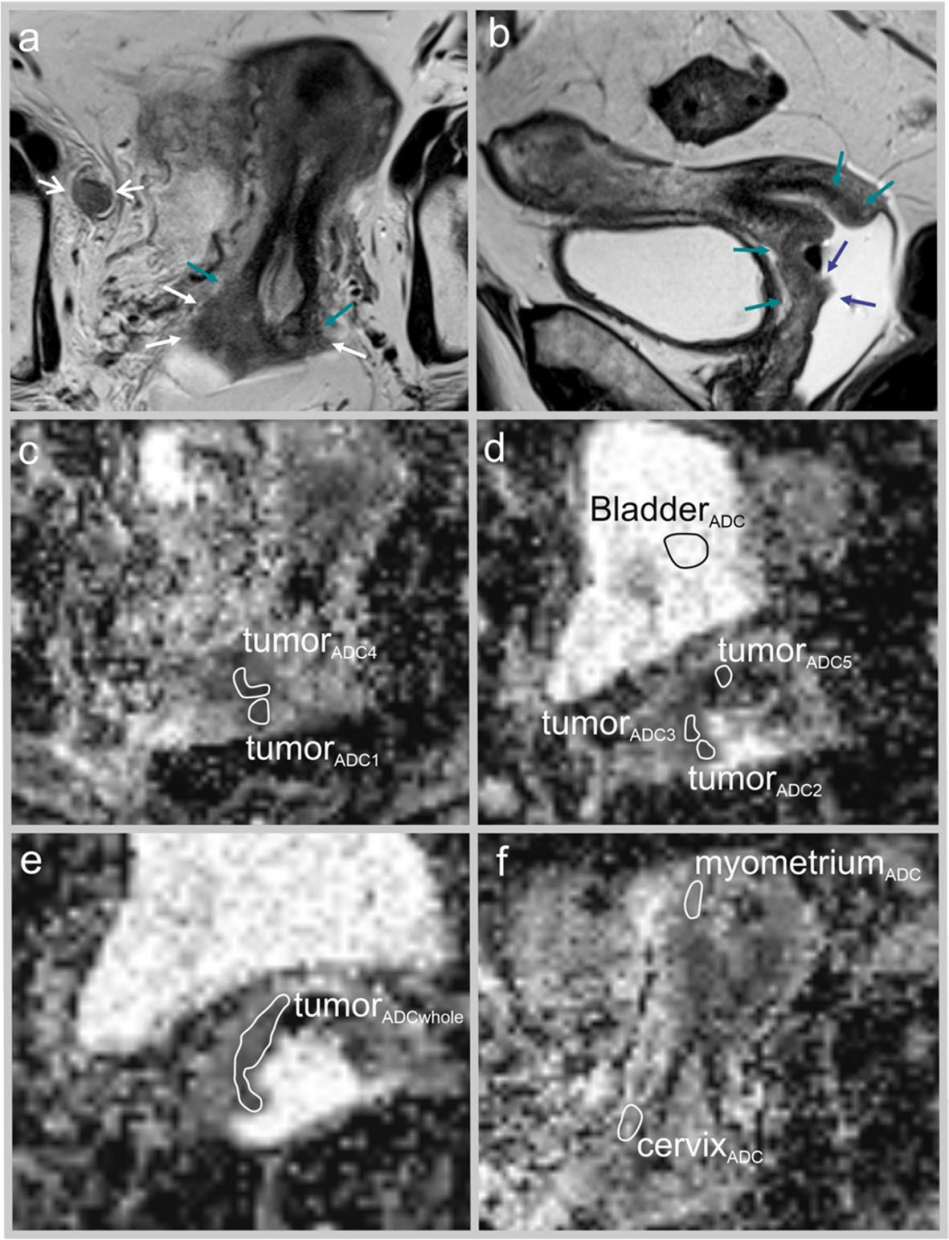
MRI in a 66-year-old woman presenting with a moderately large cervical tumor (squamous cell carcinoma, FIGO (2018) stage IIIC1) prior to treatment with concomitant radio- and chemotherapy. This patient had high myometrium_ADC_/tumor_ADCmean_-ratio and eventually died from cervical cancer 4.5 years after primary diagnosis. Axial oblique (relative to the long axis of the cervix) (a) and sagittal (b) T2-weighted MRI depicts an infiltrative moderately large (maximum tumor diameter of 3.9 cm) tumor (green arrows; a,b)in the uterine cervix with disrupted stromal ring to the right indicating parametrial invasion (white arrows; a), tumor growth into the upper 2/3 of the vagina (blue arrows; b) and enlarged right-sided iliac lymph node (white open arrows; a). Para-axial ADC-maps (c-f) depict restricted diffusion in the primary tumor. Regions of interest were drawn on the the ADC maps: tumor_ADC1–5_, tumor_ADCwhole_ bladder_ADC_, cervix_ADC_ and myometrium_ADC_ and tumor_ADCmean_ was calculated as the mean of tumor_ADC1–5_, derived by two independent readers
